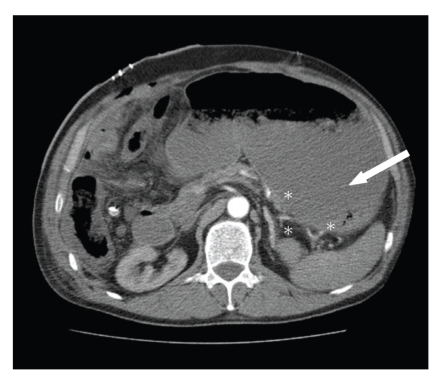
Contrast-enhanced computed tomography scan performed 8 days postoperatively (Case 1). The stomach (white arrow) is filled with blood after upper gastrointestinal bleeding from gastro-oesophageal varices secondary to portal hypertension. Multiple large collateral veins run along the stomach and spleen (white asterisk).
